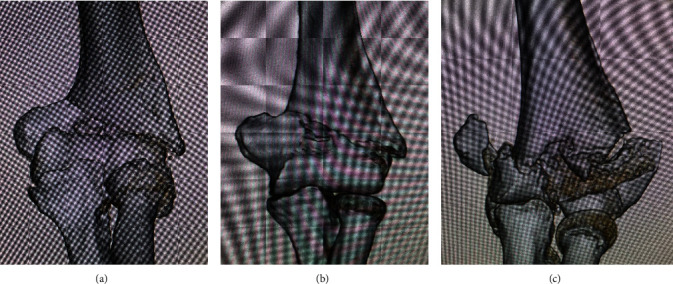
Three-dimensional computed tomography images at the time of injury: (a) patient 1; (b) patient 2; (c) patient 3.
